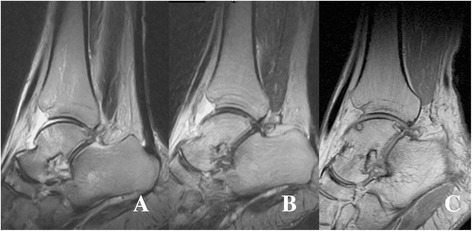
a Magnetic resonance imaging scan obtained 6 months after surgery shows high intensity at the anterior aspect of the talar body, which was caused by the vascular insufficiency. b After 12 months, the suspected lesion of talus had disappeared and had returned to nearly the normal signal compared with adjacent bones. c After 5 years, the reimplanted talus had a small-sized subchondral lesion without osteonecrosis
